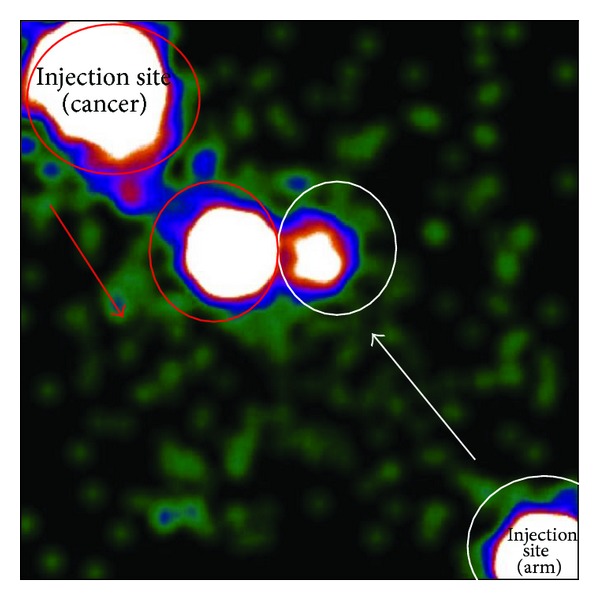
Intraoperative lymphoscintigraphy (^99m^TC-labelled human albumin microcolloid, subdermally injection) of patient with palpable left breast cancer (red circles) 1 h after previous axillary reverse mapping through a lower dose of radiotracer injected subcutaneously in the intramuscular groove of inner ipsilateral arm (white circles). Anterior view shows the progressive acquisition of two different patterns of lymphatic drainage carried out in separate times.
